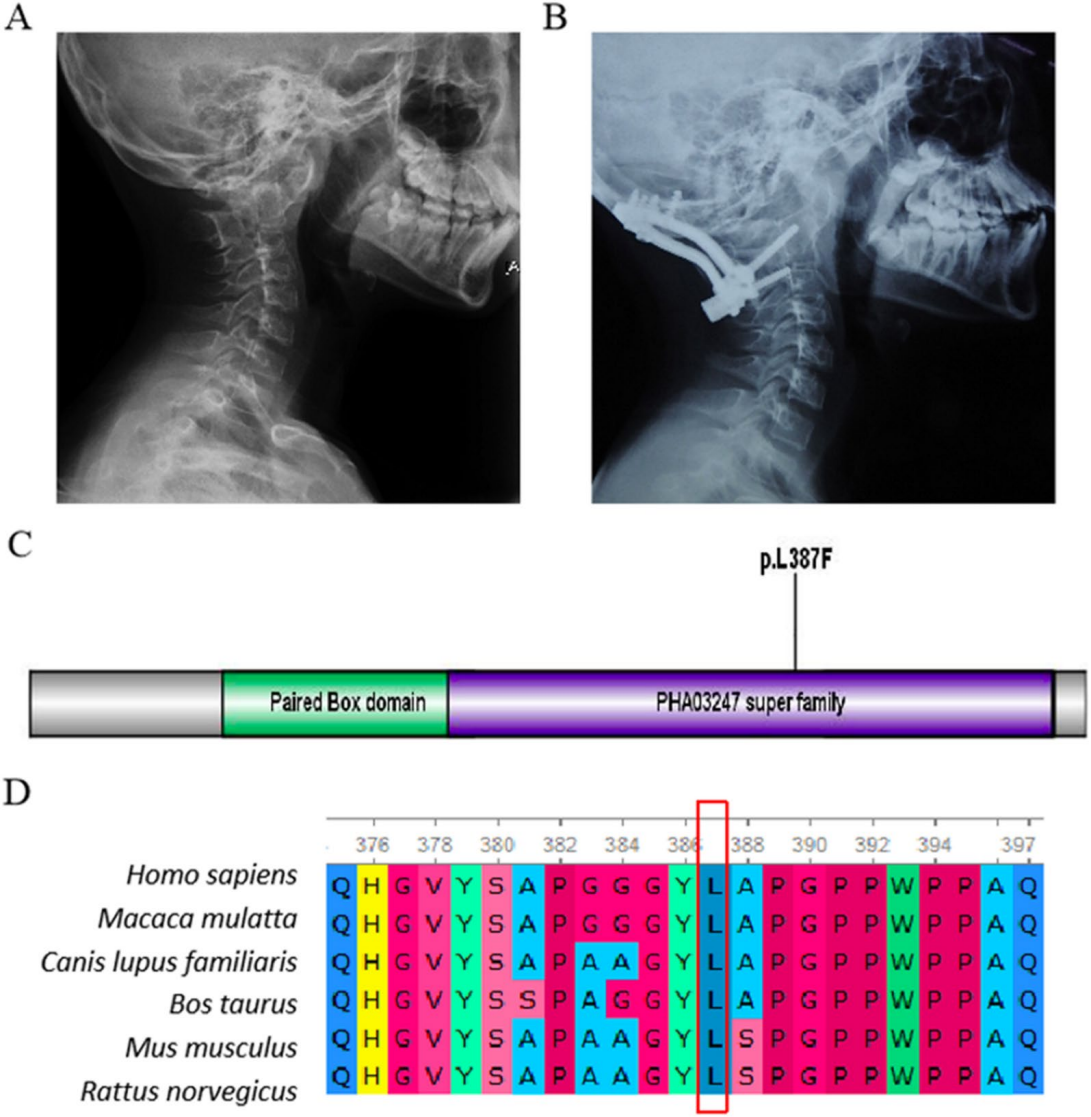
The analysis of variants c.555G > A (p.K185K) and c.1159C > T (p.L387F) in *PAX1* in Patient 19. A Pre-operative lateral X-ray of the cervical spine demonstrating the classic “sandwich fusion” and atlantoaxial dislocation. B Post-operative lateral X-ray of the cervical spine at the one-year follow-up demonstrating maintenance of reduction of atlantoaxial dislocation by occipito-cervical fixation consisting of six occipital screws, one C2 pedicle screw, and one C3 pedicle screw. C Conserved Domain Search was performed, and the variant c.1159C > T (p.L387F) was located in the PHA03247 superfamily domain of the protein. D Multiple sequence alignments revealed that the mutation site in PAX1 was highly evolutionarily conserved
